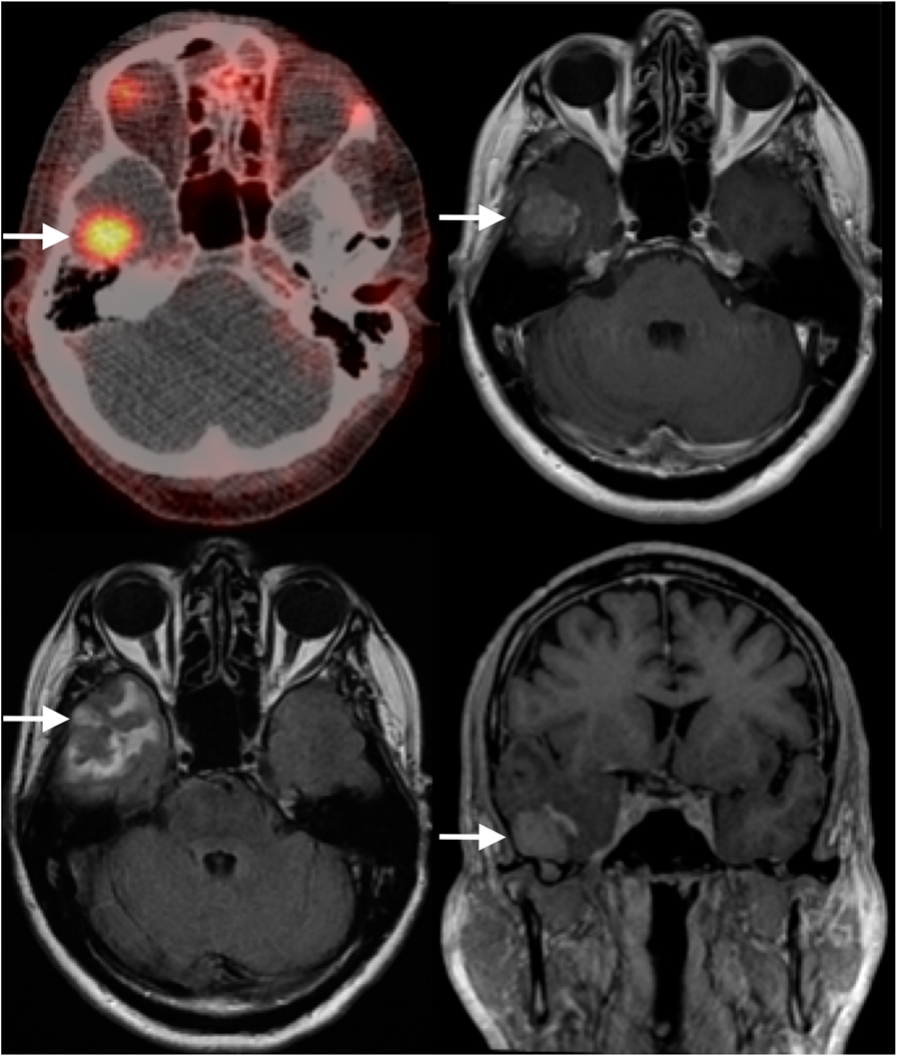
Selected images through the skull base from the [^68 Ga]Ga-THP-PSMA PET/CT and subsequent MRI. Top left: axial fused PET/CT image revealing focal uptake in the right middle cranial fossa; bottom left: axial FLAIR MRI showing white matter oedema in the right temporal lobe; top (axial) and bottom (coronal) right: post-contrast enhanced T1-weighted MR images demonstrating an enhancing lesion in the floor of the right middle cranial fossa in close contact with the dura mater
